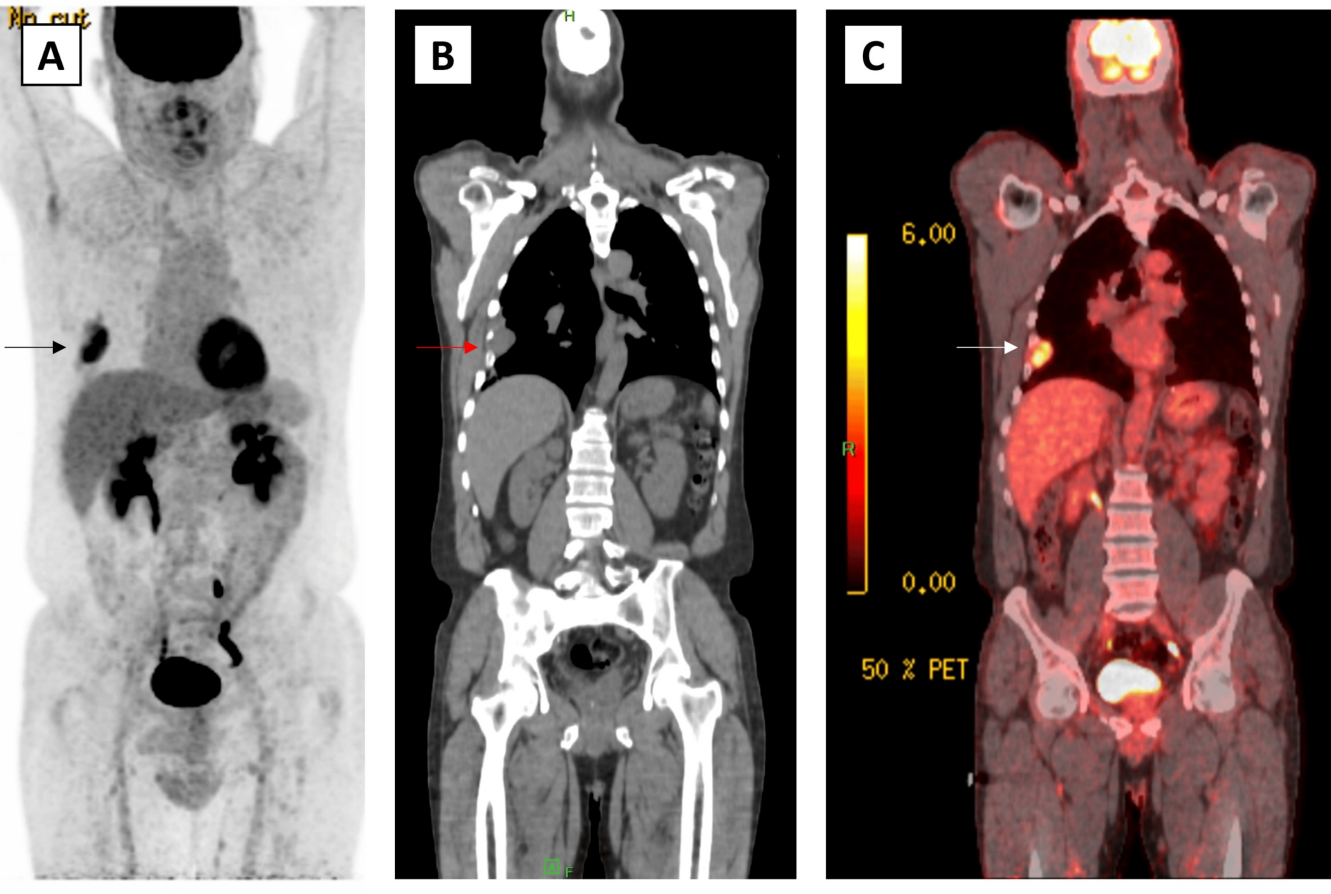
Preoperative MIP and PET/CT scans of the schwannoma to identify areas of potential metastatic spread and metabolic activity MIP: Maximum intensity projection, PET/CT: Positron emission tomography/Computed tomography; A: Coronal MIP scan, B: Coronal CT scan, C: fusion PET/CT scan, Arrows: schwannoma
